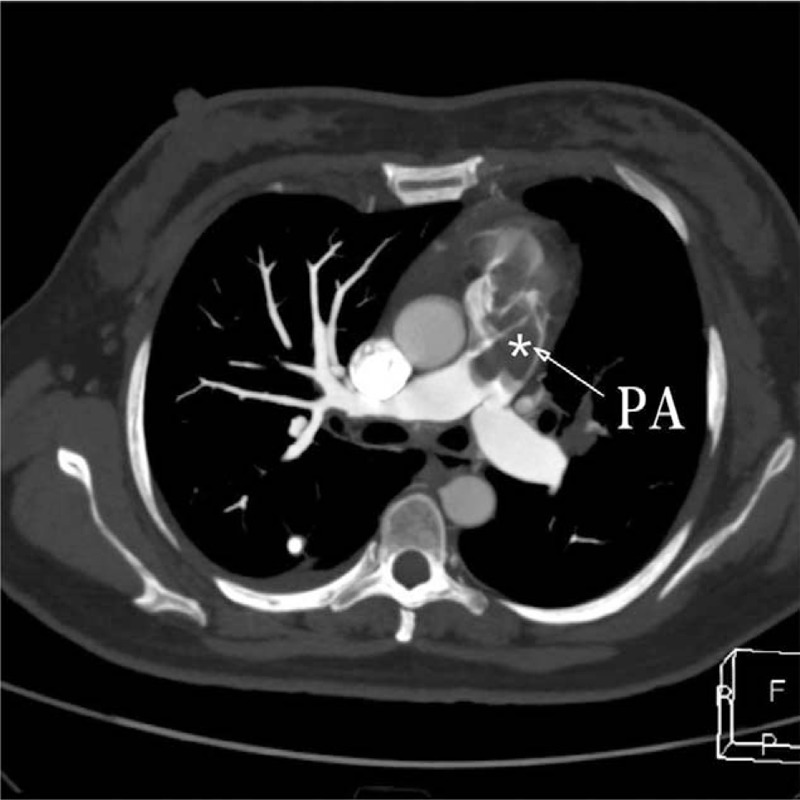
Pulmonary CTA showed that there are low density filling defects (asterisks) in the pulmonary arteries. PA = pulmonary artery.
